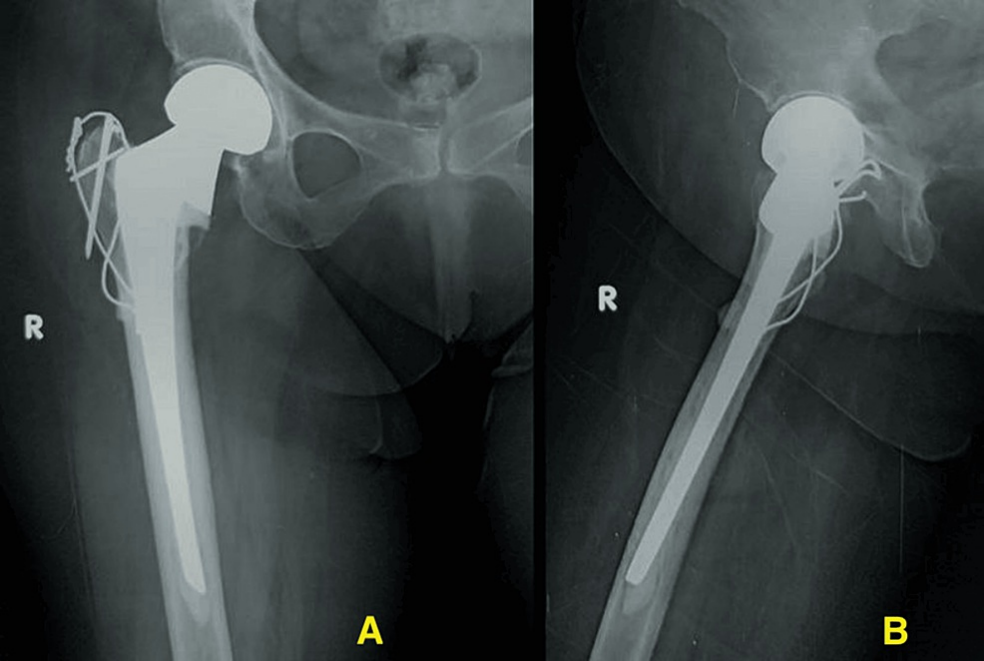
Postoperative radiographs showing cemented bipolar prosthesis and fixation of greater trochanter with tension-band wiring 9A: Anteroposterior view of the right hip with thigh showing cemented bipolar prosthesis with tension-band wiring of the greater trochanter. 9B: Lateral view of the right hip with thigh
